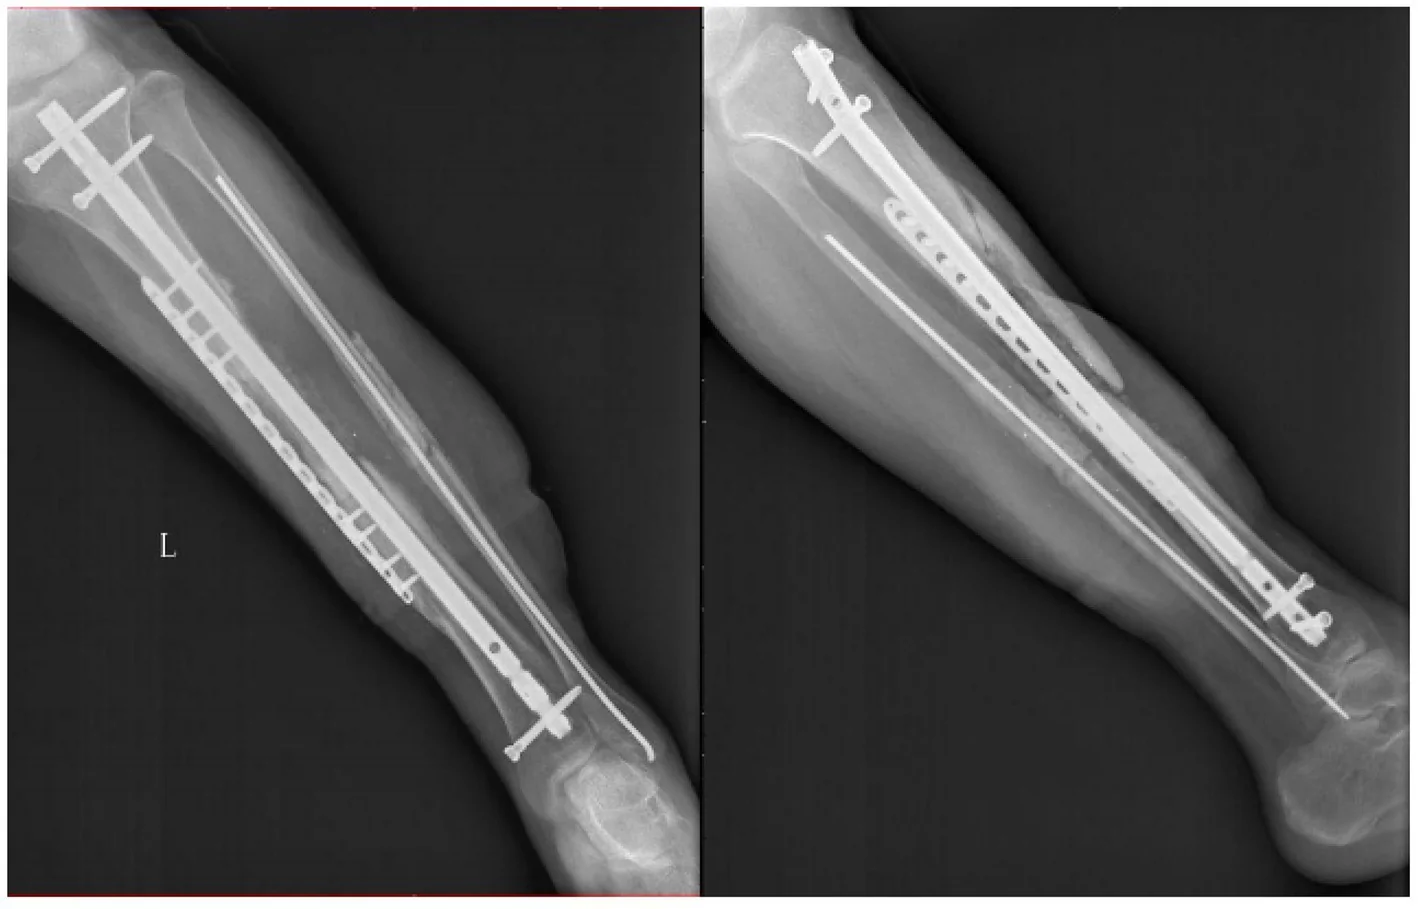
The X-ray findings two months after the bone grafting procedure, revealing partial bone resorption in the grafted area.
